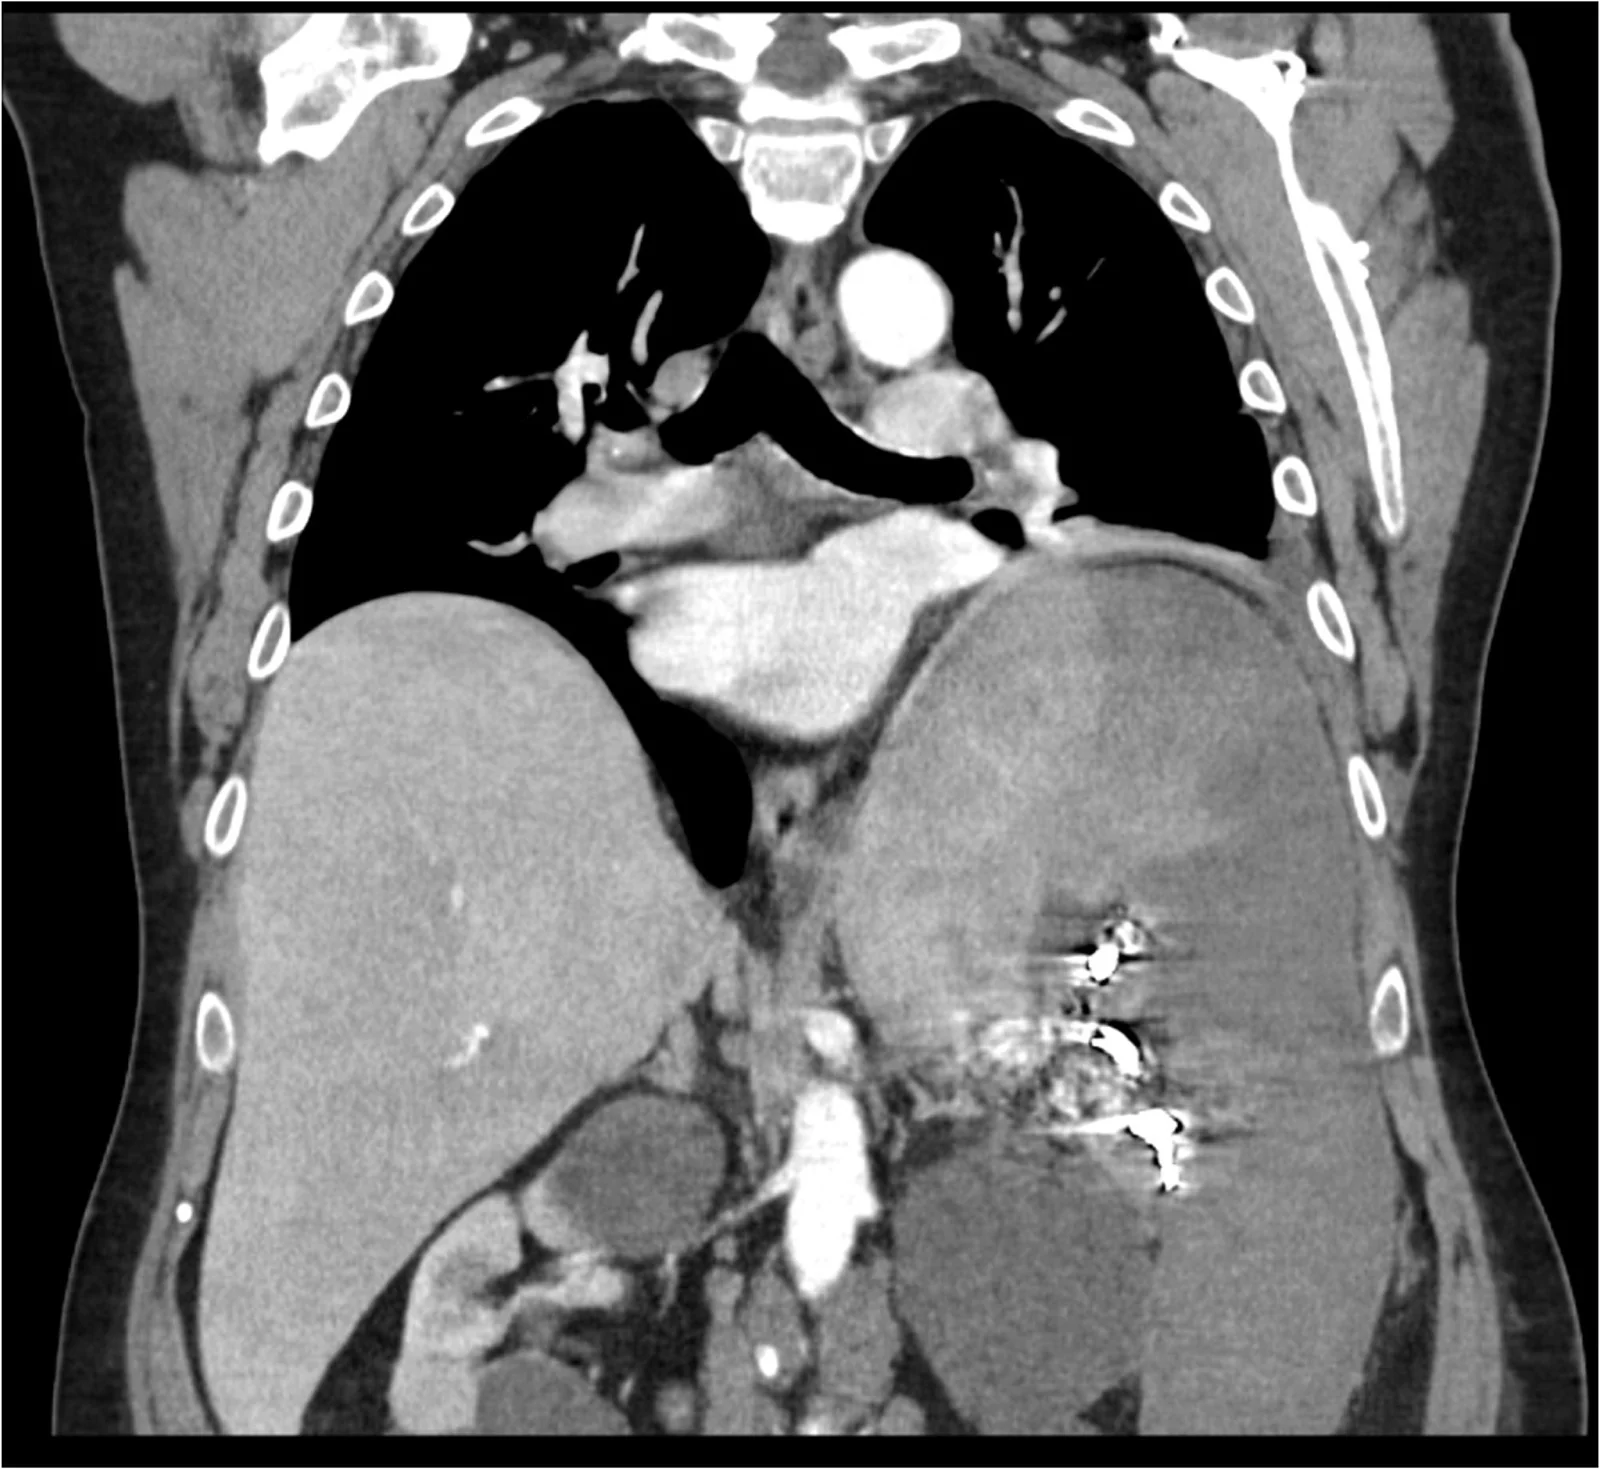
Follow up CT chest with contrast coronal view 21 days post embolization procedure shows continued expected evolving post embolization changes to the spleen including volume reduction and hypodense nonenhancement.
